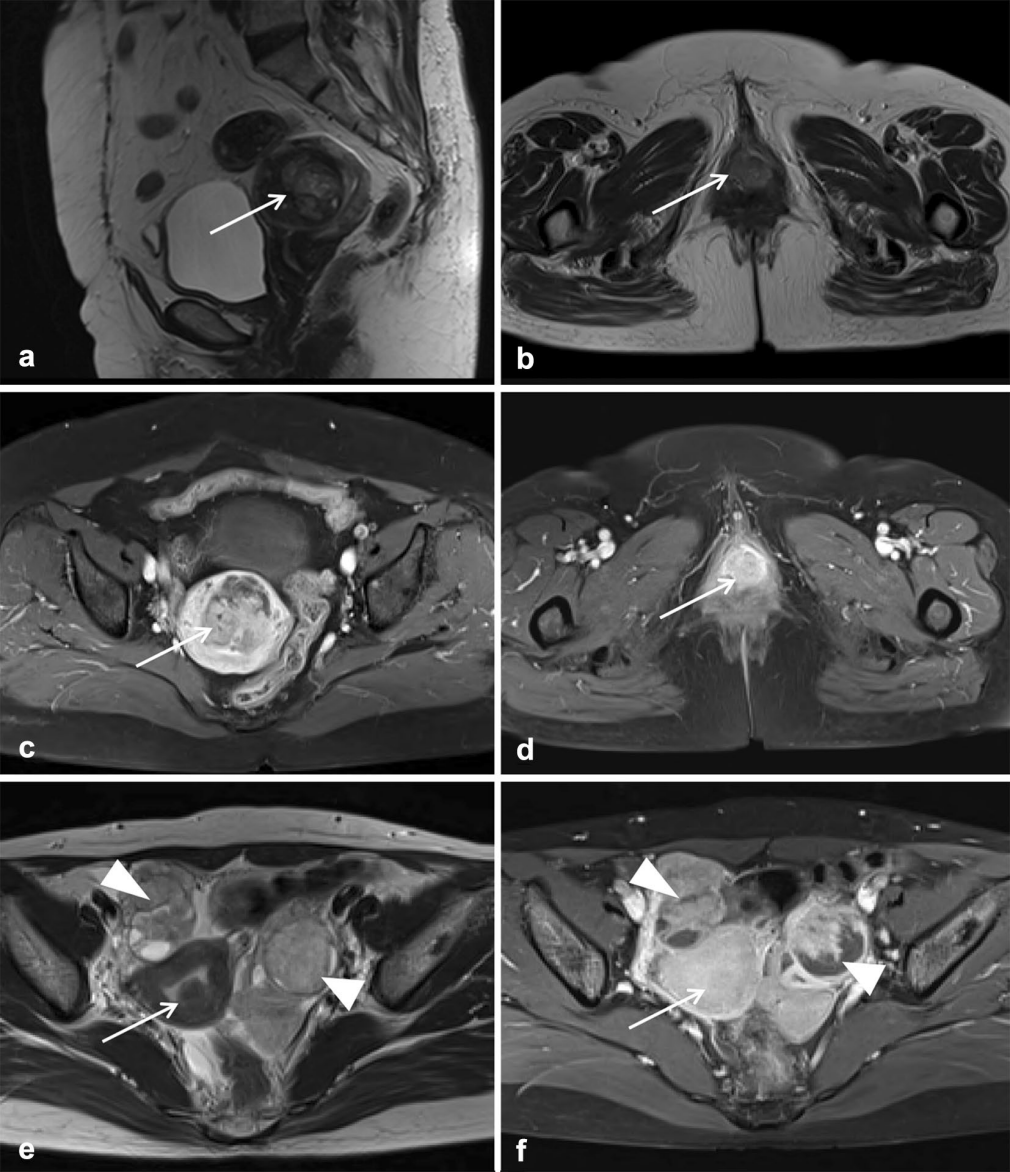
Preoperative pelvic MRI findings of malignant uterine PEComa (case 1, a–d). Sagittal T2-weighted (a) and axial T2-weighted (b) images showed two masses with heterogeneous hyperintensity in the uterine myometrium (*arrow* in a) and the lower vagina (*arrow* in b). Axial fat-suppressed contrast-enhanced T1-weighted images (c, d) showed the enhancement of the masses (*arrows* in c, d). Preoperative pelvic MRI findings of benign uterine PEComa (case 2, e, f). Axial T2-weighted (e) and fat-suppressed contrast-enhanced T1-weighted (f) images showed a well-defined, homogeneous submucosal uterine mass (*arrows*), showing signal intensity or enhancement similar to that of the myometrium. Note bilateral ovarian complex cystic masses with solid enhancing portions (*arrowheads*)
